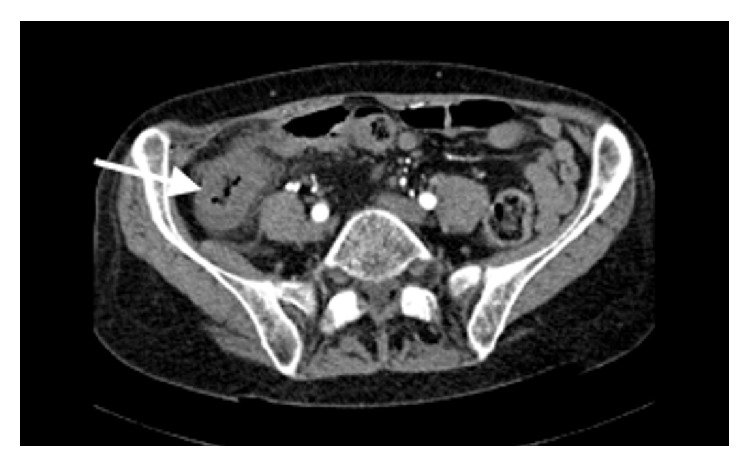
Abdominal CT scan with contrast demonstrating inflammatory changes of the pericolonic fat in the right colon particularly around the cecal area.
